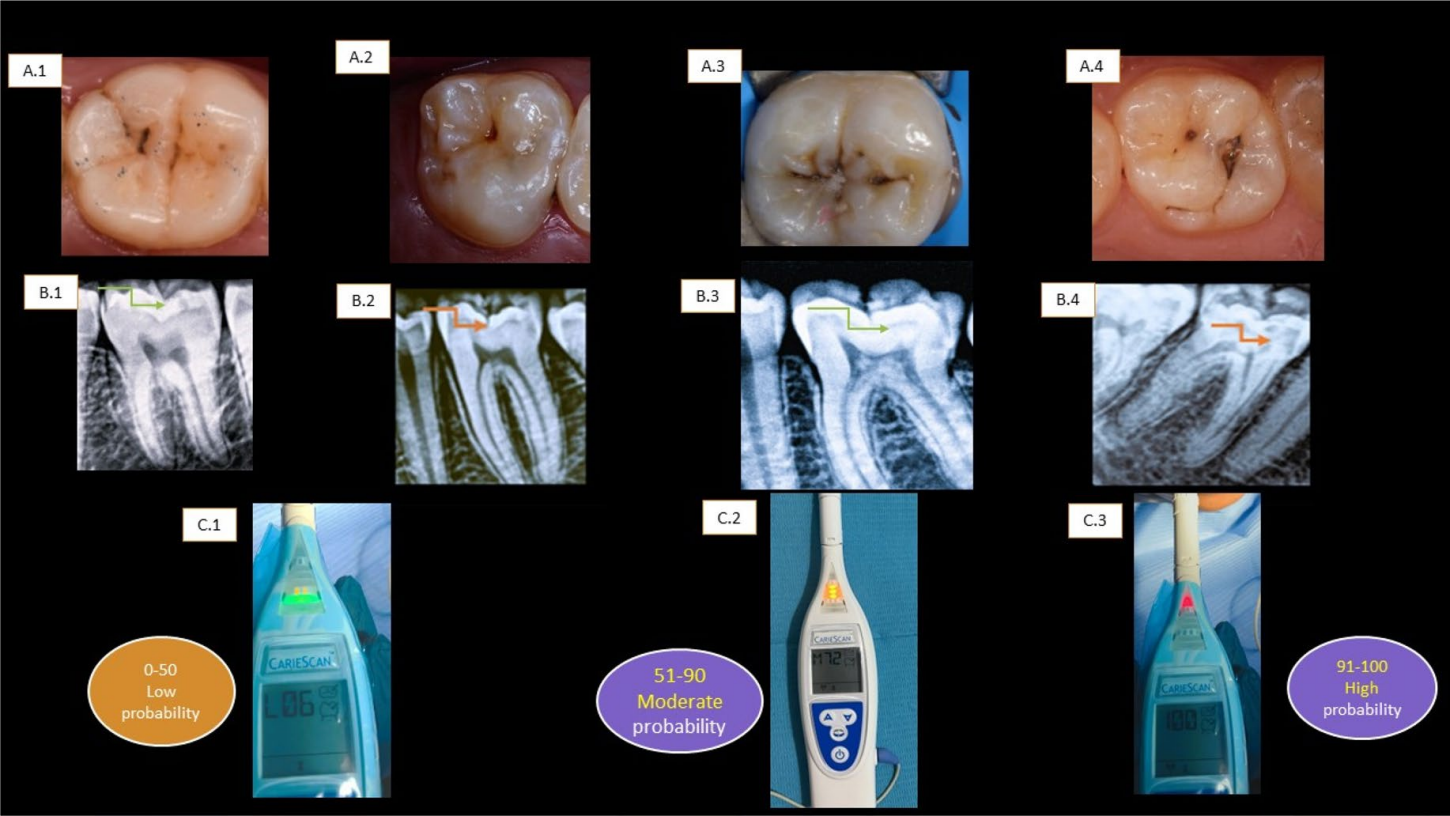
Shows different ICDAS-II scores (A) with its corresponding periapical digital radiographs (B) and CariesScan Pro scores (C). (A.1) Clinical Photo representing ICDAS score 1. (A.2) Clinical Photo representing ICDAS score 2. (A.3) Clinical Photo representing ICDAS score 3. (A.4) Clinical Photo representing ICDAS score 4. (B.1) Digital periapical radiograph showing occlusal caries in lower molar in the outer half of enamel with score R1 (red arrows). (B.2) Digit al periapical radiograph showing occlusal caries in lower molar in the inner half of enamel with score R2 (red arrows). (B.3) Digital periapical radiograph showing occlusal caries in lower molar in outer third of dentin with score R3 (red arrows). (B.4) Digital periapical radiograph showing occlusal caries in lower molar in middle third of dentin with score R4 (red arrows) dentin with score R4 (red arrows). (C.1) scores 0–50 with green led light resulting in a low probability, (C.2) a score ranging from 51 to 90 with yellow led light resulting in a moderate probability, (C.3) a score ranging from 91 to 100 with red led light resulting in a high probability of the presence of caries.
